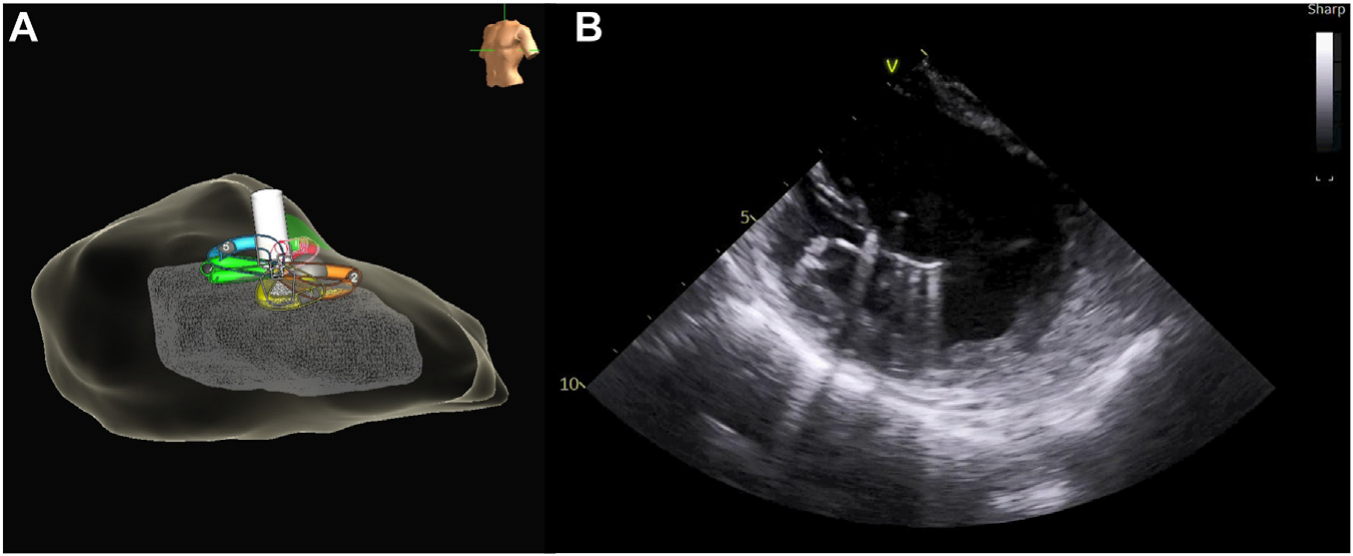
A: Farawave pulse field ablation (PFA) ablation catheter in flower configuration sitting on top of the posteromedial papillary muscles (PM). B: Intracardiac echocardiography (ICE) showing the Farawave PFA ablation catheter in flower configuration sitting on top of the posteromedial PM before ablation.
